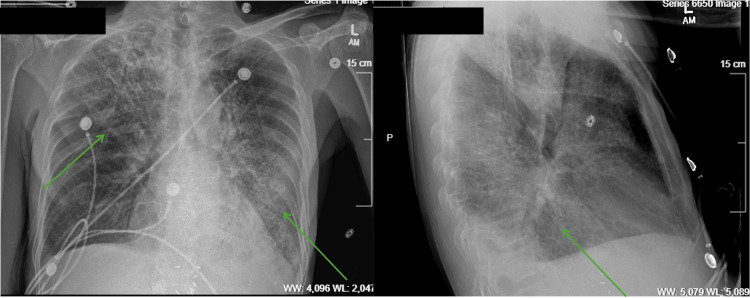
Two-view chest radiograph on day four exhibiting worsening bilateral infiltrate with ground-glass appearance (green arrows).
